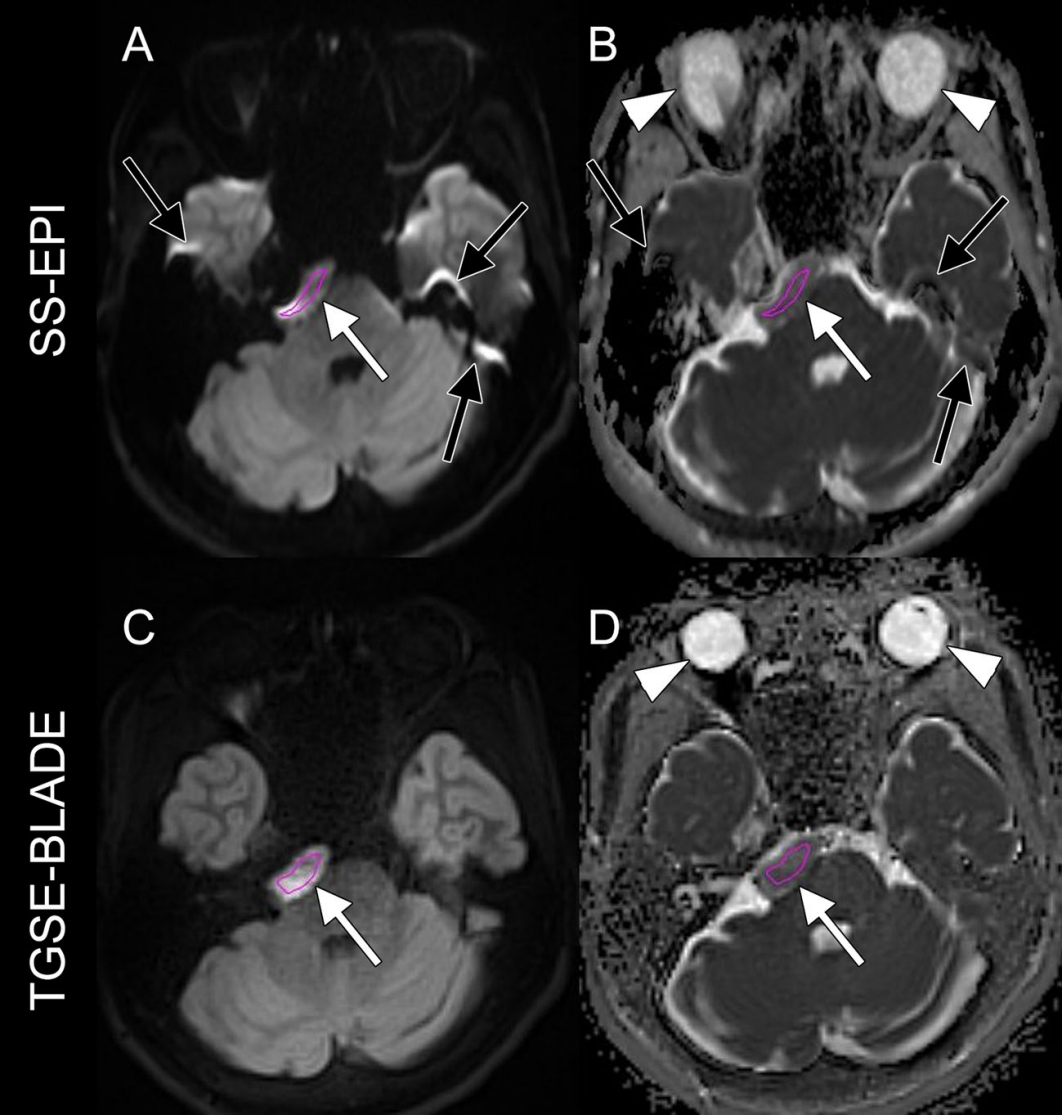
Another rater selected a representative slice in which the lesion was well recognized on both DWI techniques, and carefully placed medium-size ROIs for the epidermoid cyst while avoiding artifacts on b1000 images using SS-EPI and TGSE-BLADE DWI (white arrows) A, C) Juxta-marginal ROIs. These ROIs were used for ADC calculation (white arrows, B, D). Note that multiple susceptibility artifacts are shown on SS-EPI DWI (black arrows, A, B). The shape of the eyeballs is deformed on SS-EPI (arrowhead, B), but remains circular on TGSE-BLADE (arrowhead, D)
